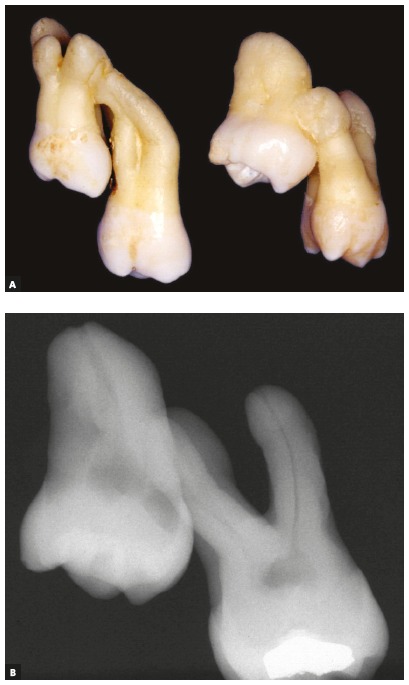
Second and third molars joined by cement, in teeth with and without hypercementosis. Radiographic imaging does not allow root individualization in teeth isolated from other tissues; in vivo, this individualization tends to be even harder.
